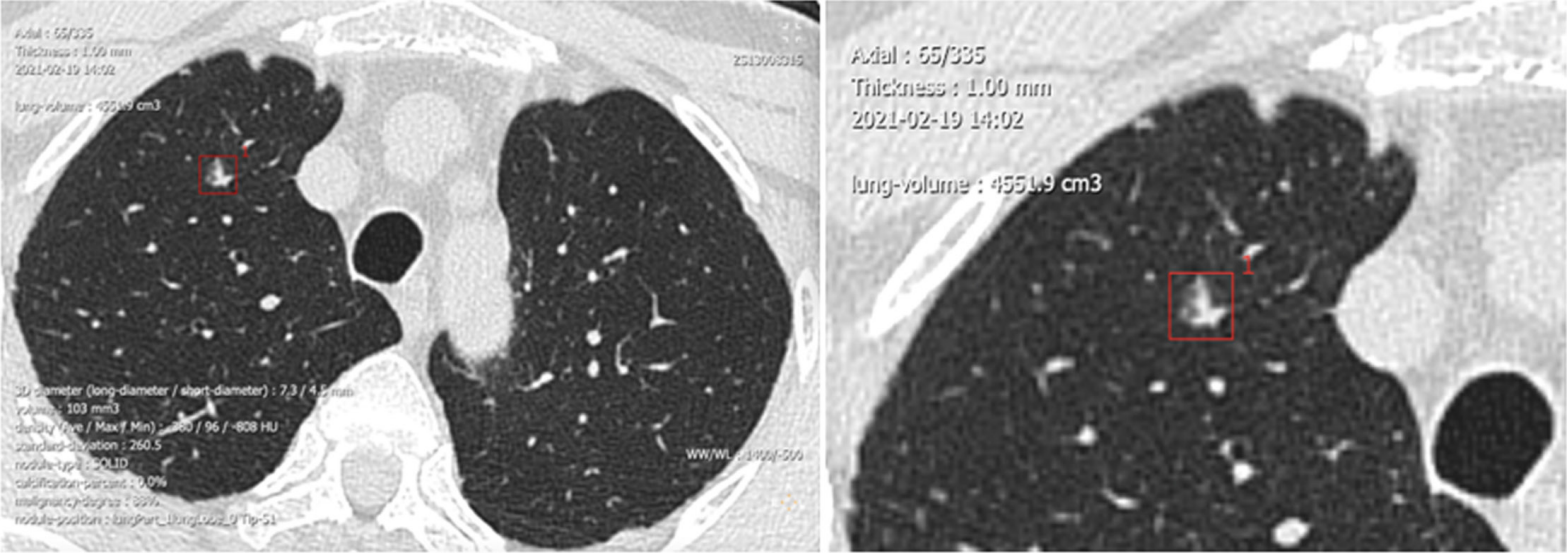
A ground‐glass opacity found in the right upper lung by routine computed tomography (CT).
